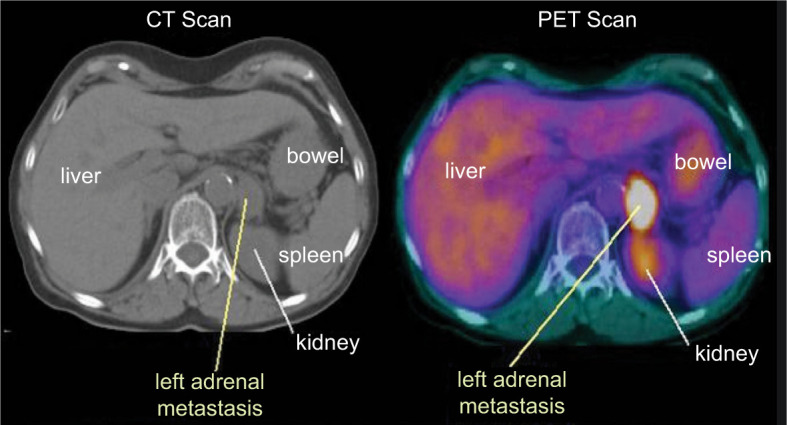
Computed tomography and positron emission tomography imaging showing adrenal gland metastasis.
